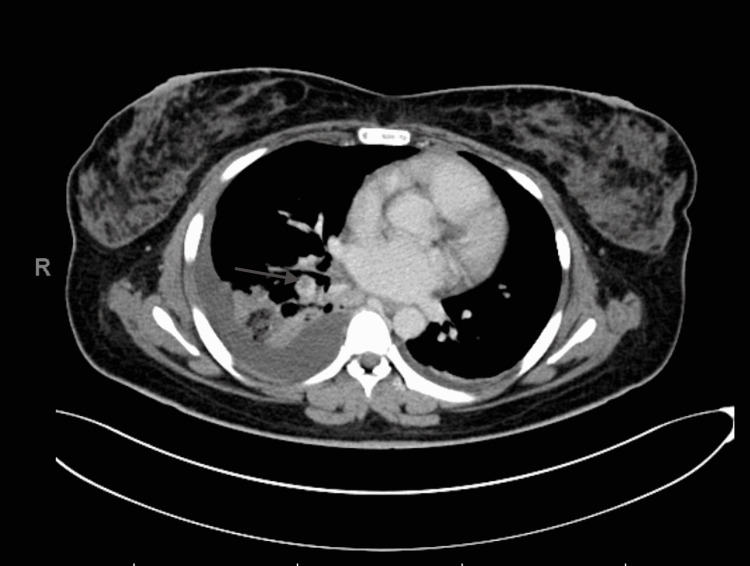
Transverse CT chest view shows a filling defect in the sub-segmental branches of the pulmonary arteries on the right side representing pulmonary embolism associated with consolidation and bilateral pleural effusion (more over the right side)
